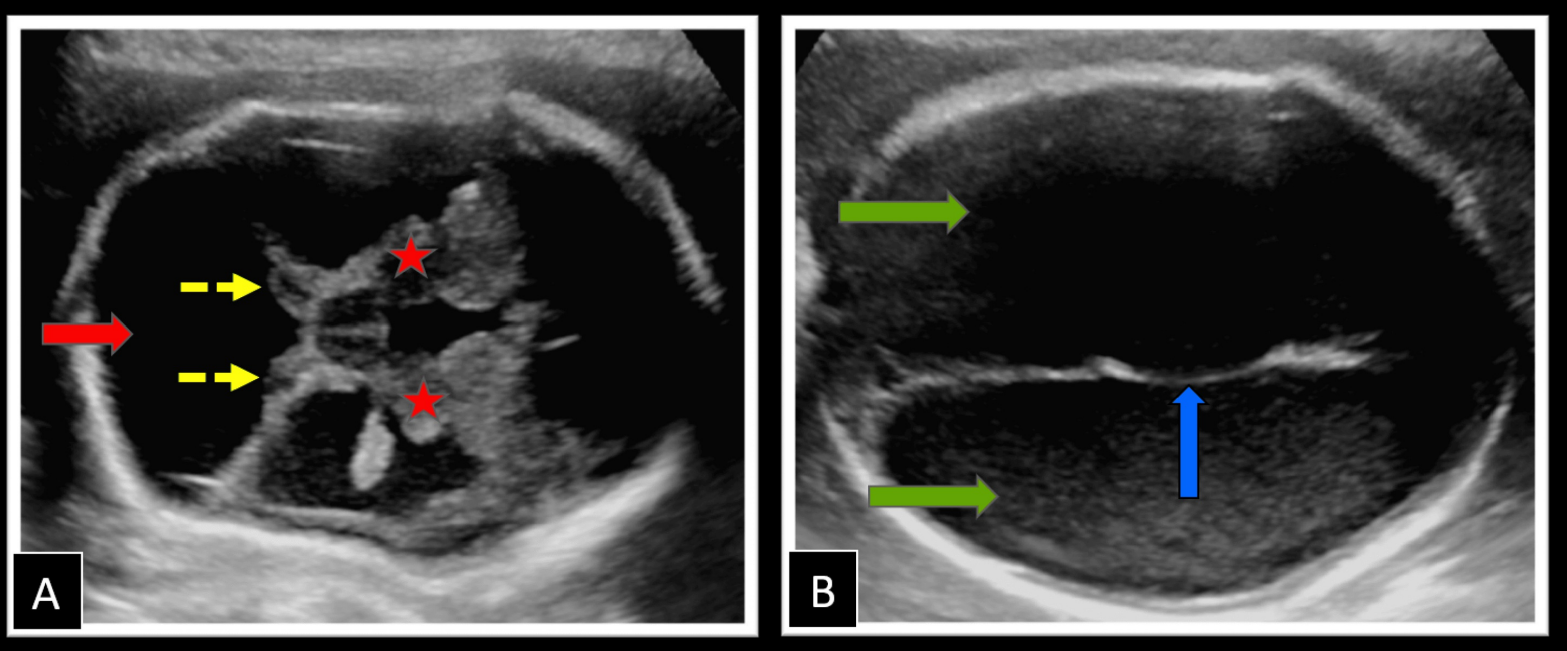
Ultrasound examination of the fetal central nervous system showing the presence of Dandy-Walker malformation (A) Transverse ultrasound image of the fetal brain showing an enlarged posterior fossa with a cerebrospinal fluid containing cyst (red arrow) communicating with the fourth ventricle, with hypoplastic bilateral cerebellar hemispheres (yellow arrows) with vermian aplasia. Also note the normal thalami (stars) with no evidence of fusion and a dilated third ventricle between them. (B) Transverse section through the fetal skull revealing bilateral dilated lateral ventricles (green arrows) with a normal midline falx (blue arrow).
